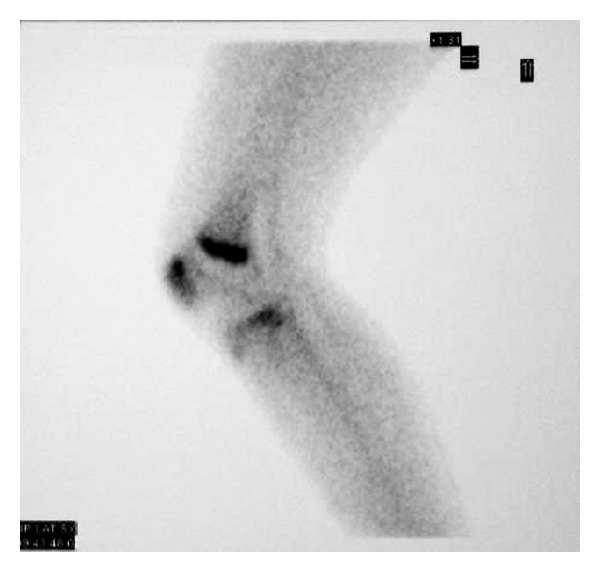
Three-phase scintigraphy showing a picking up area on the superior part of the patella.
